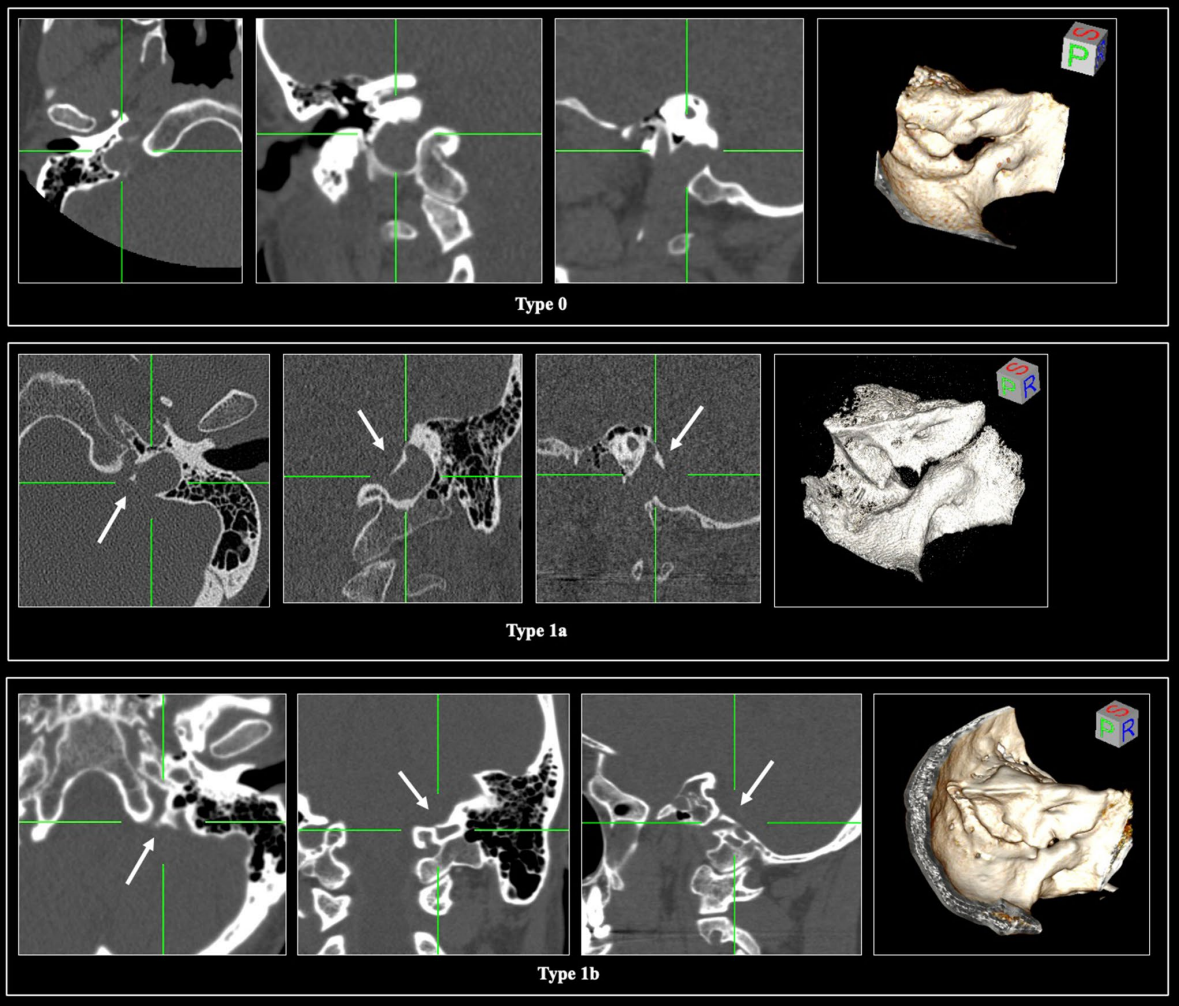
The morphology of the jugular foramen (JF) using computed tomography scans. The presence of the intrajugular process (IJP), classified as type 1, is clearly depicted in axial, coronal, and sagittal slices based on its morphology, whether partial (type-1a) or complete (type-1b). In contrast, the absence of the IJP is designated as type 0
